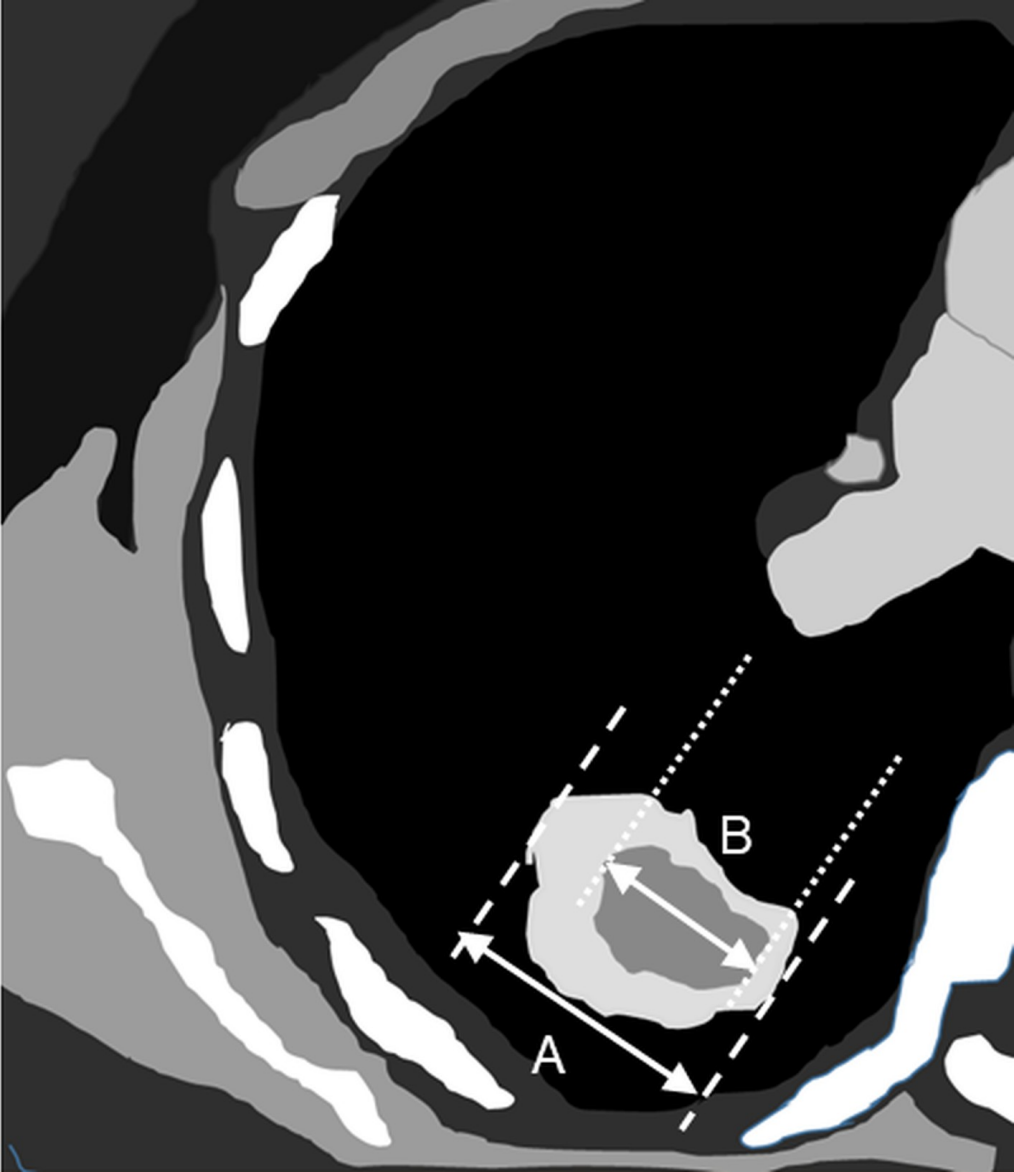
Definition of consolidation tumor ratio (B/A). A: Maximal diameter of the consolidation part of the tumor was measured in CT cross section under lung window view. B: Maximal diameter of the consolidation part of the tumor was measured in CT cross section under mediastinal window.
